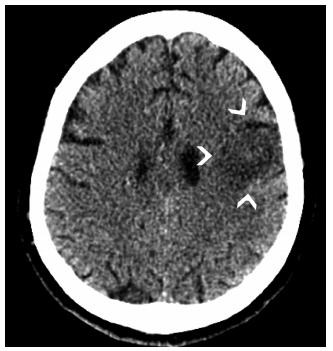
Patient 6. Axial unenhanced computed tomography - ischemic stroke acute findings compromising cortical and subcortical white matter of the left middle frontal gyrus (arrowheads).
